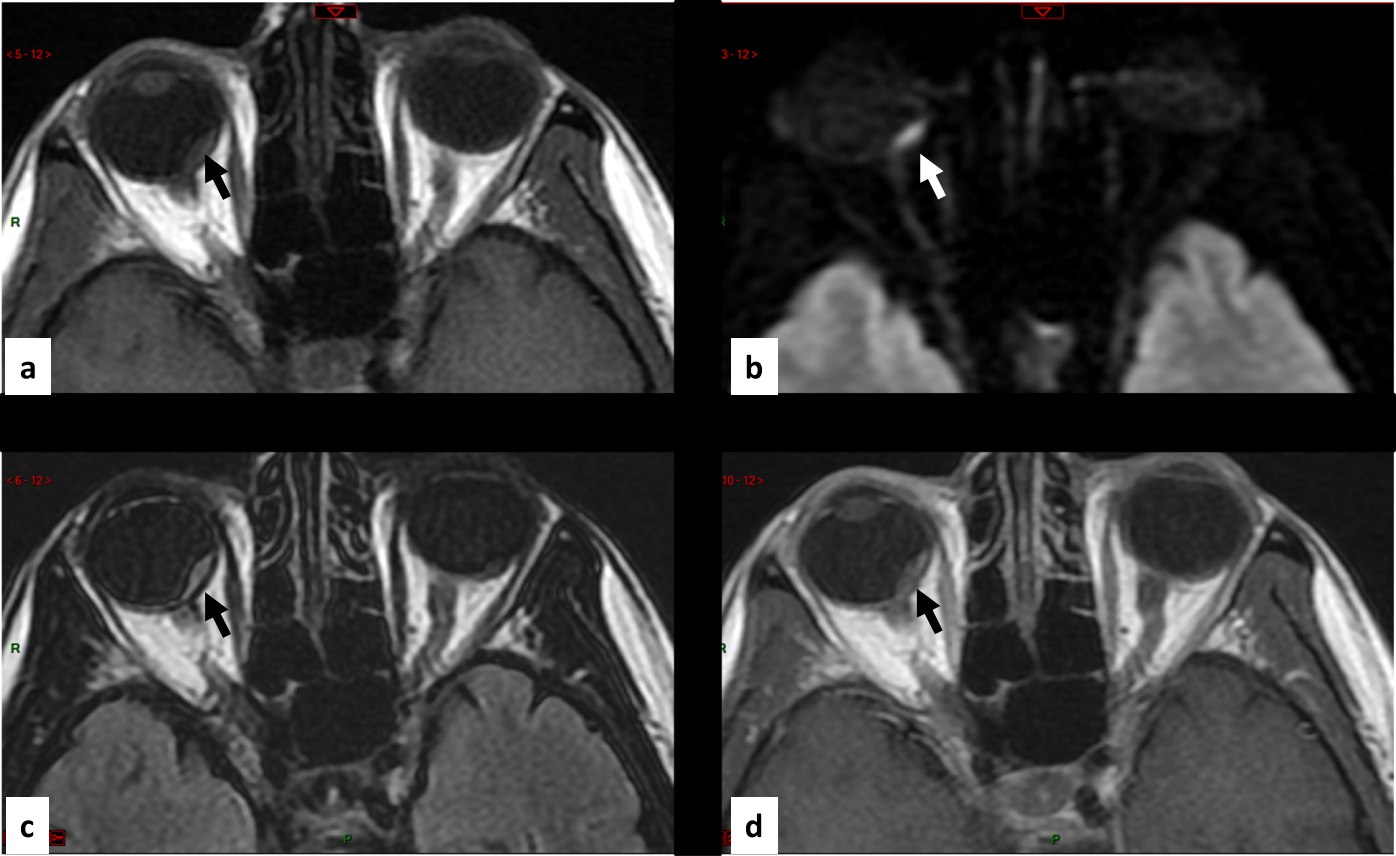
MRI In axial plane focused on orbits with the following sequences in Patient #7. *)* Spin-Echo T1-weighted image, it shows an oblong intraorbital lesion (arrow) in slight hypersignal within the orbit. b Diffusion-weighted image, it shows a frank hypersignal of the lesion (arrow). c FLAIR, it shows the lesion in slight hypersignal (arrow). d Spin-Echo T1-weighted image after contrast injection, it shows a slight enhancement of the intraorbital lesion (arrow)
